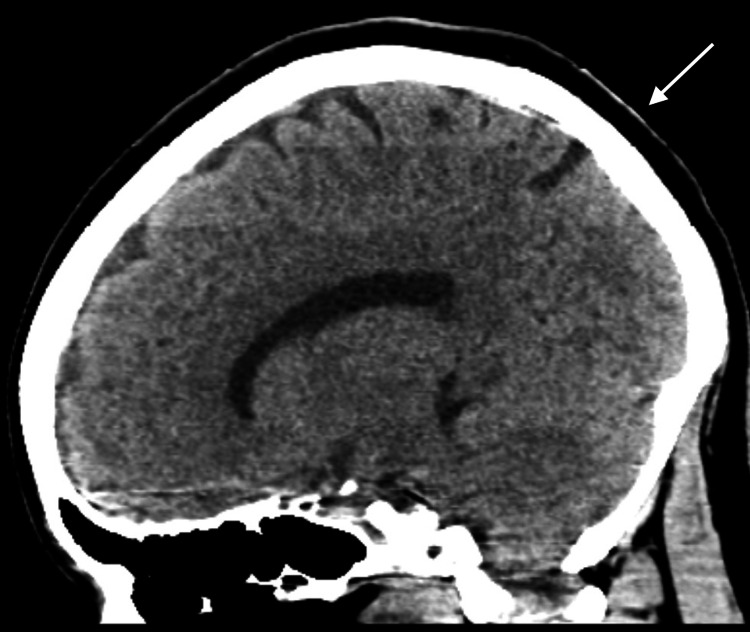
CT scan of the head (sagittal view) with no evidence of acute hemorrhage, ischemia, or mass CT: computed tomography
